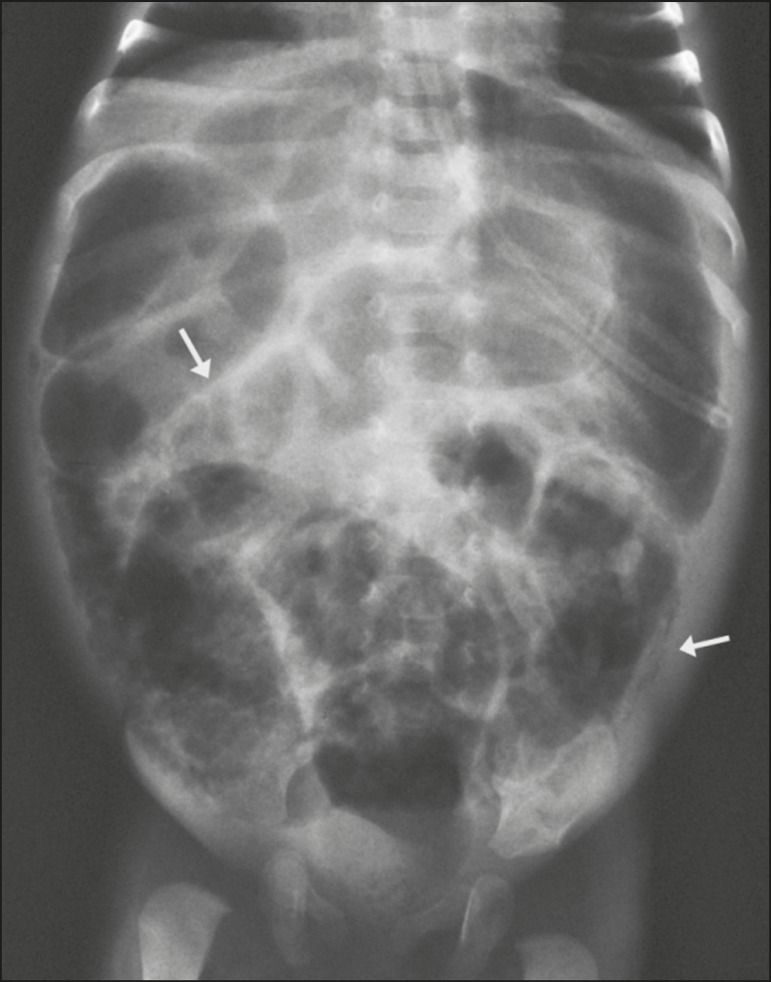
X-ray of a preterm newborn, in the supine position with vertical
